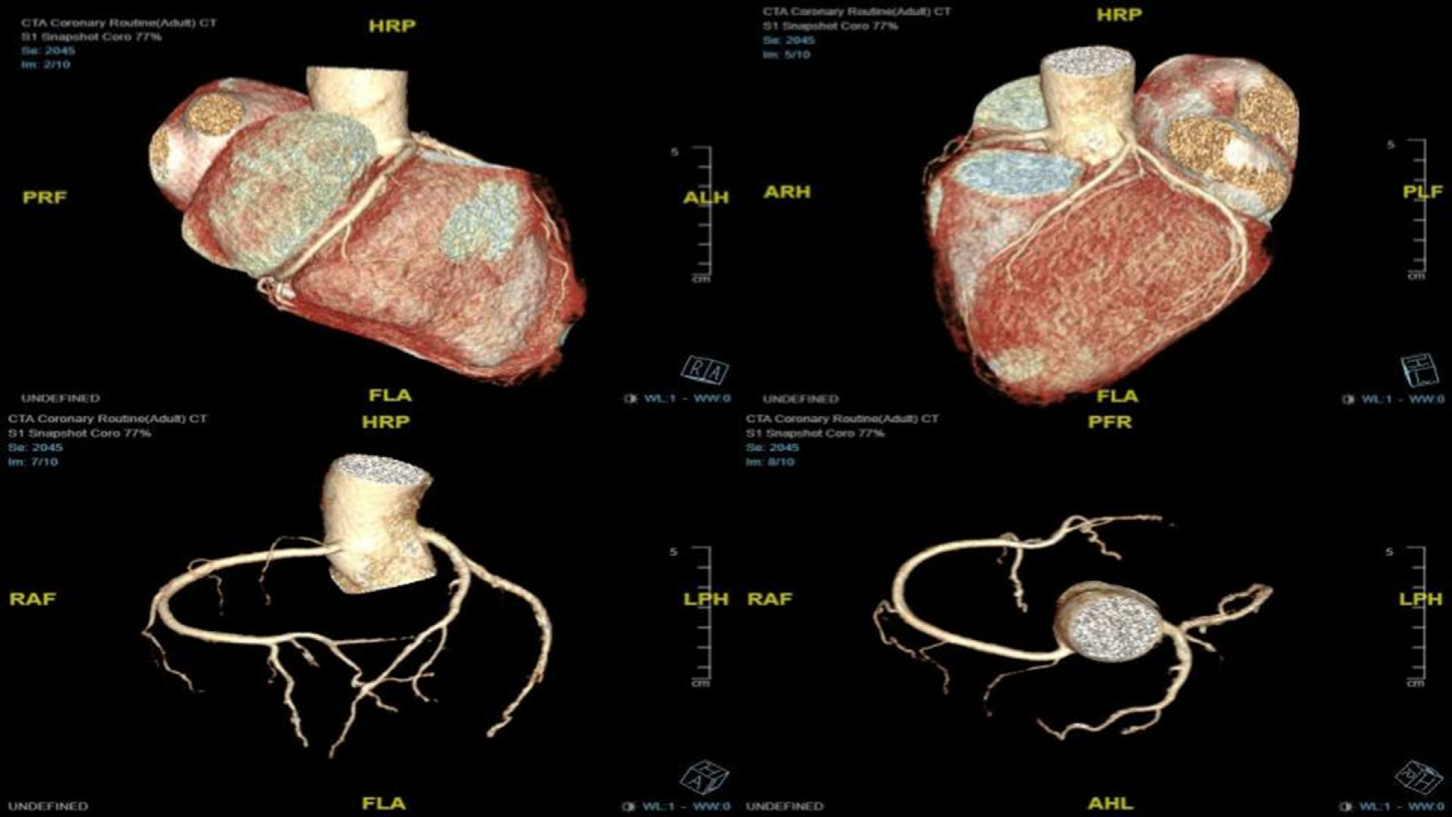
Coronary Computed Tomography Angiography Revealed Normal Coronary Anatomy AHL = anterior head left; ALH = anterior left head; ARH = anterior right head; FLA = feet left anterior; HRP = head right posterior; LPH = left posterior head; PRF = posterior right feet; PLF = posterior left feet; RAF = right anterior feet.
